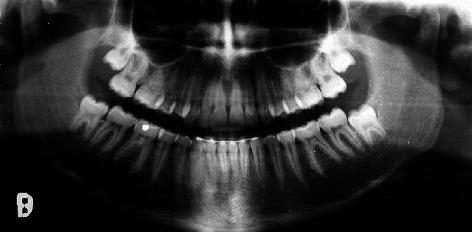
2-year follow-up panoramic radiograph.
